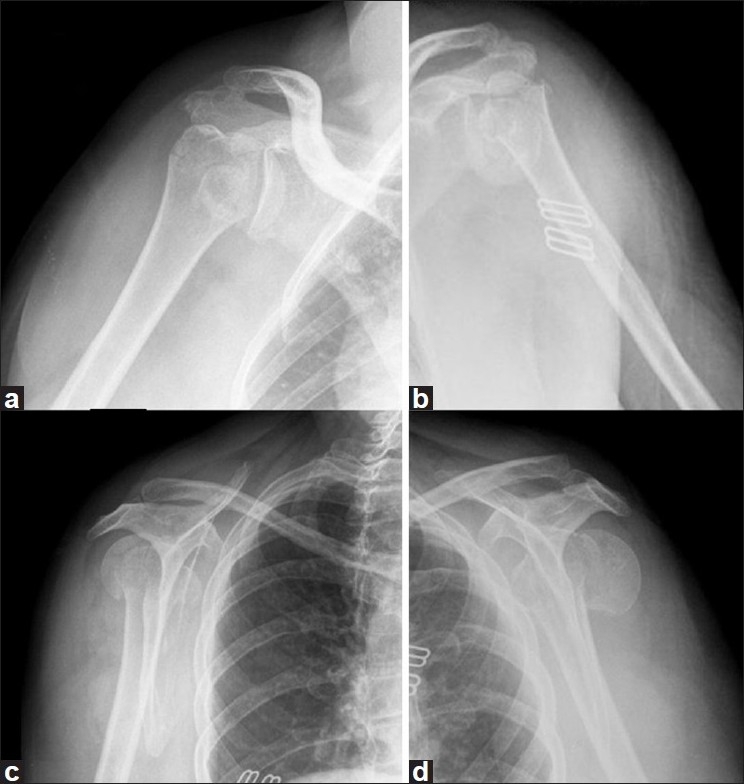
Radiographs of both shoulders [right- (a,c); left- (b,d)] showing a bilateral posterior four-part fracture-dislocation of the shoulderr
